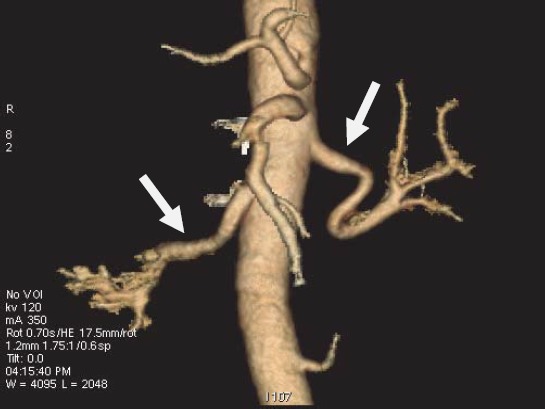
Angio-CT for the renal arteries.
